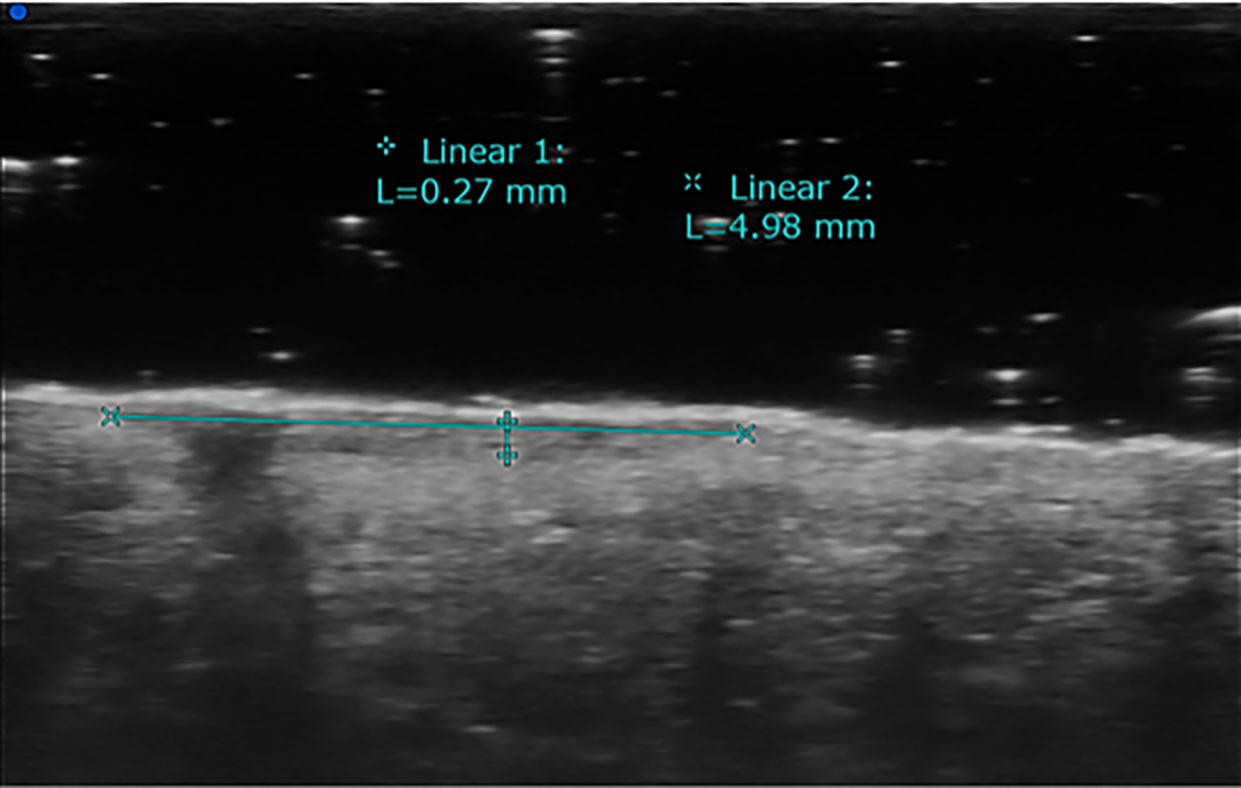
High-frequency transducers allow the determination of ultrasound Breslow index, which means evaluating the depth of tumor invasion. This example shows skin melanoma considered with HFUS (70 MHz).
